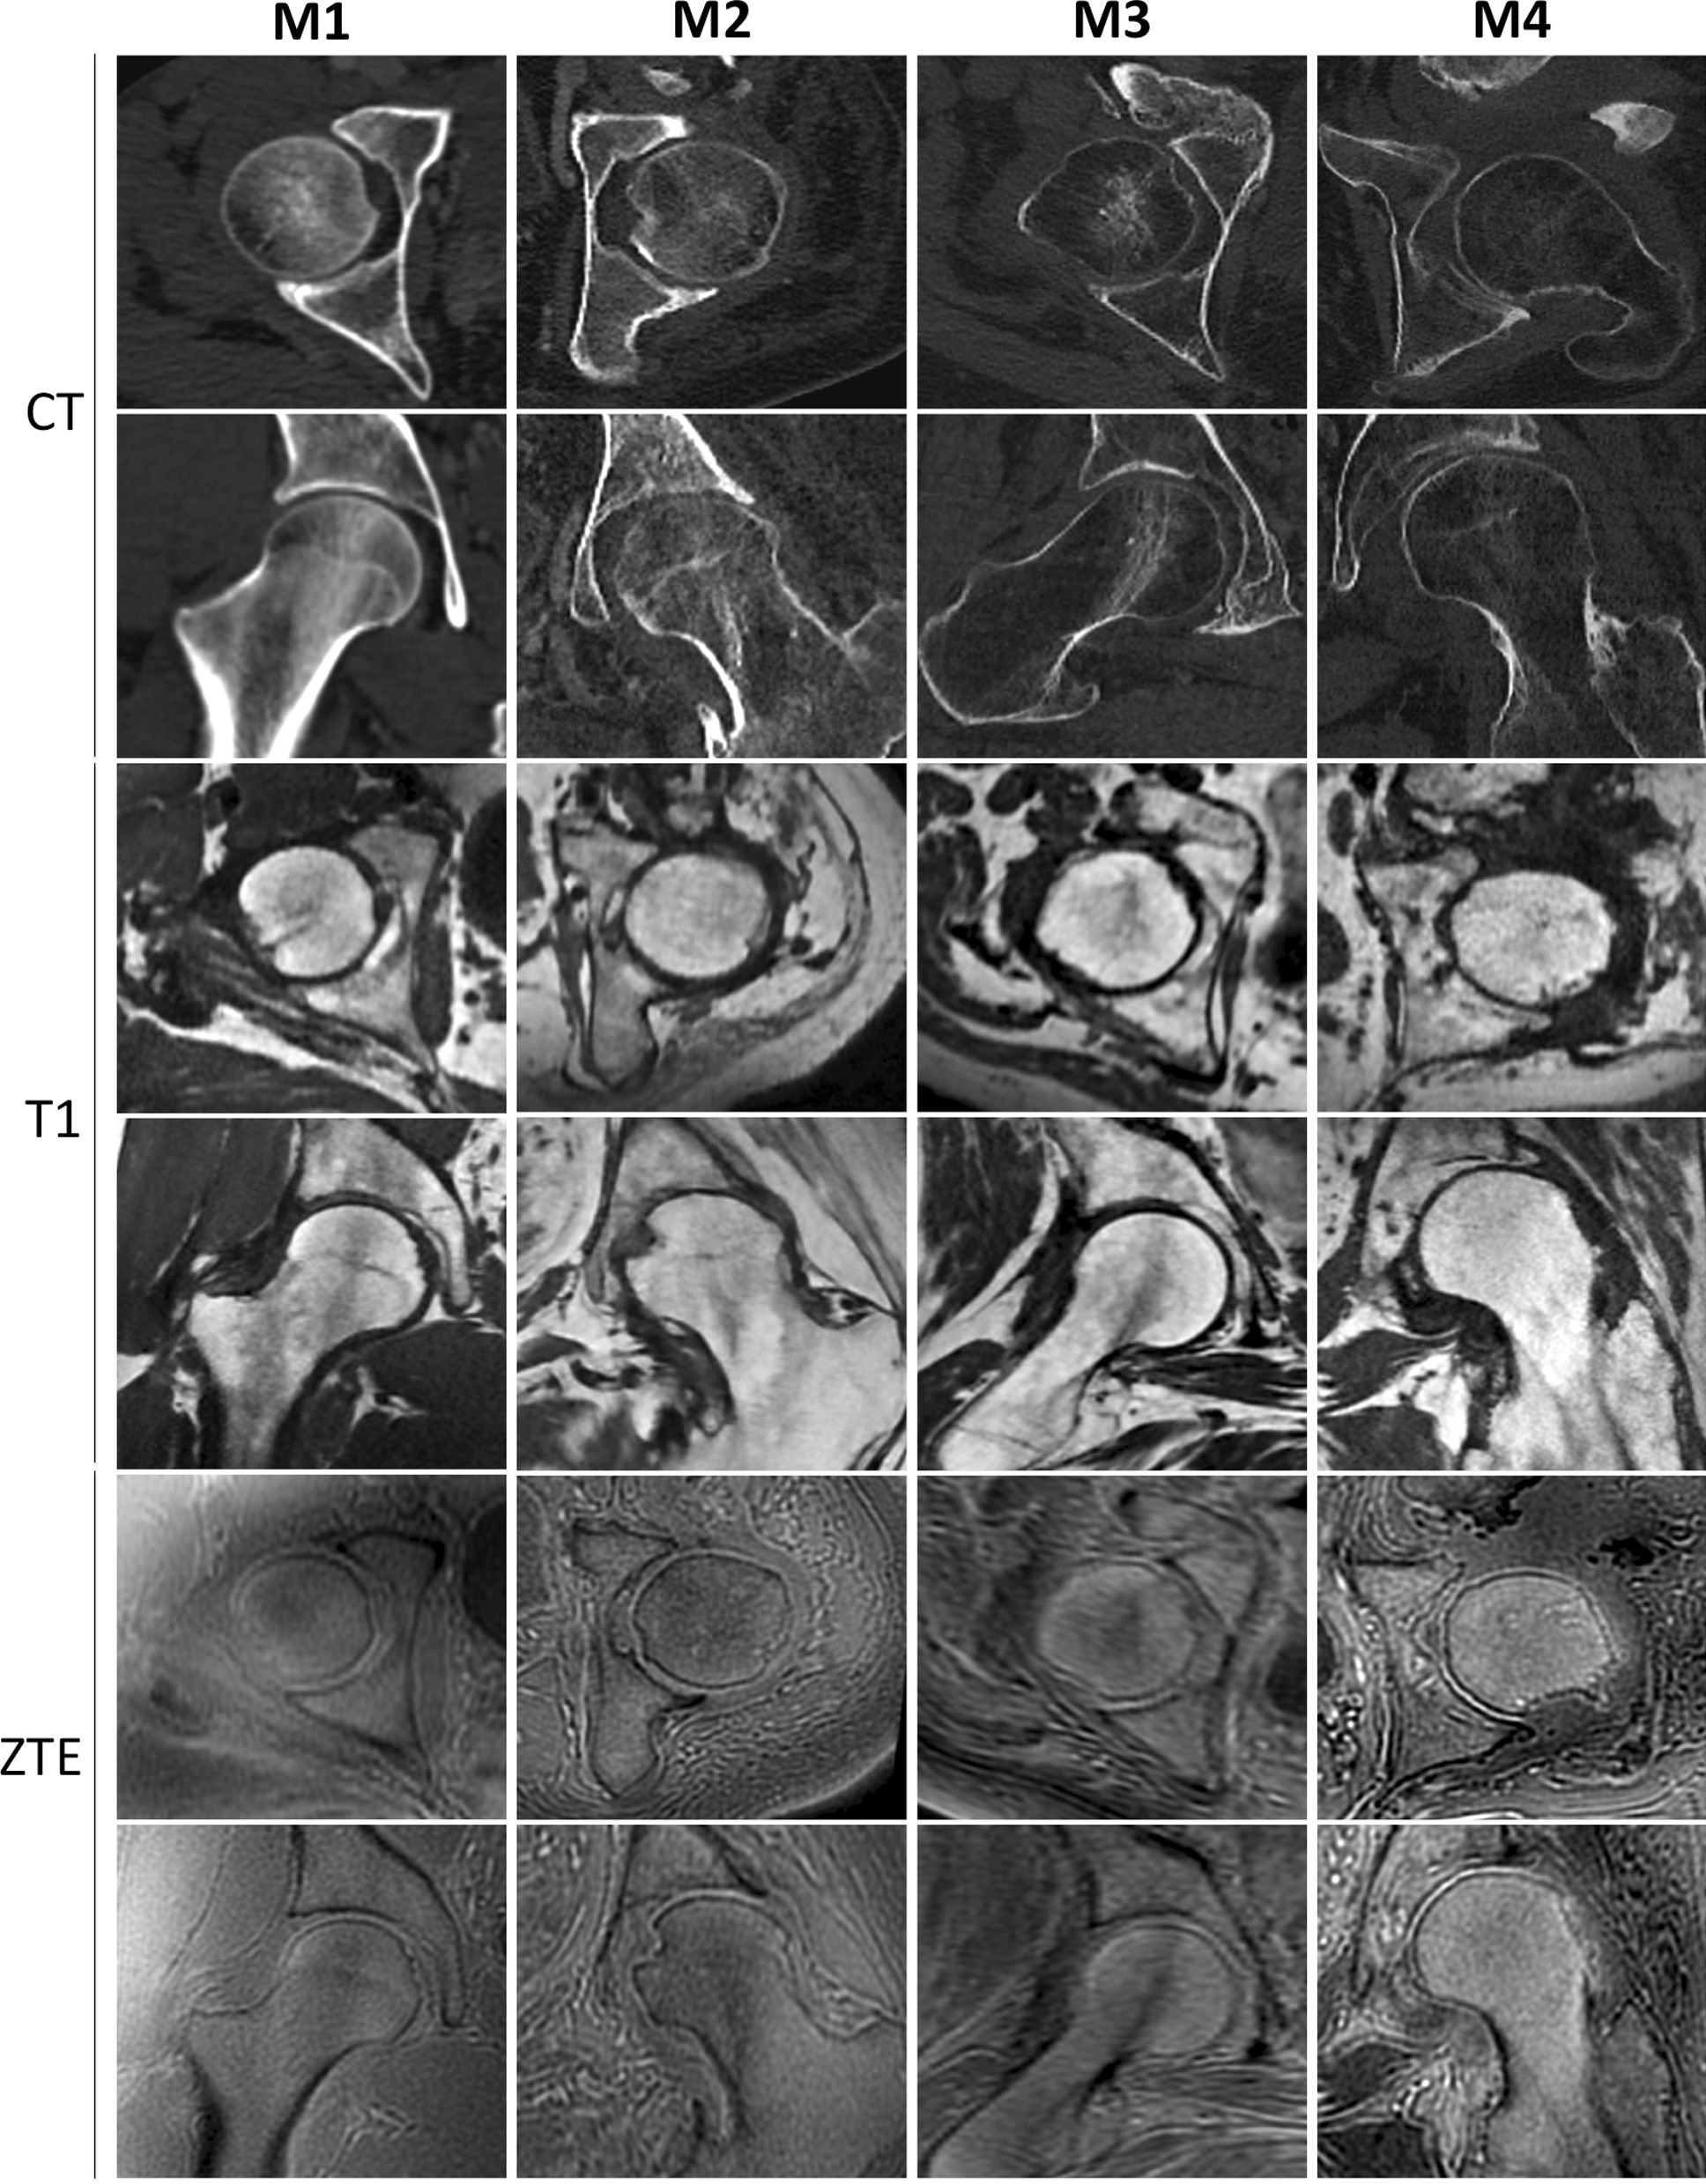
Qualitative evaluation of bone mineralization on CT and on MRI in T1-weighted and ZTE sequences, axial and coronal views of the femoral head. Each column shows a category of bone mineralization: M1, M2, M3 and M4
